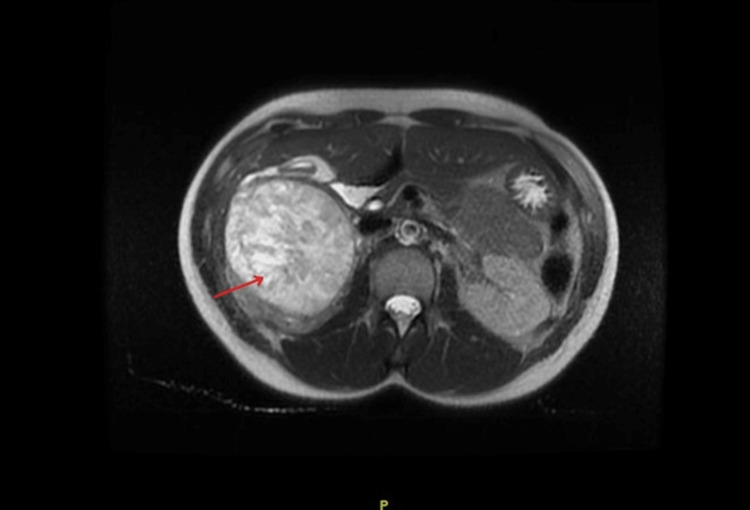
Axial T2-weighted MRI abdomen. The red arrow demonstrates a large 8 cm x 10 cm mass with heterogeneous high signal intensity in the right suprarenal/adrenal region. MRI: magnetic resonance imaging
